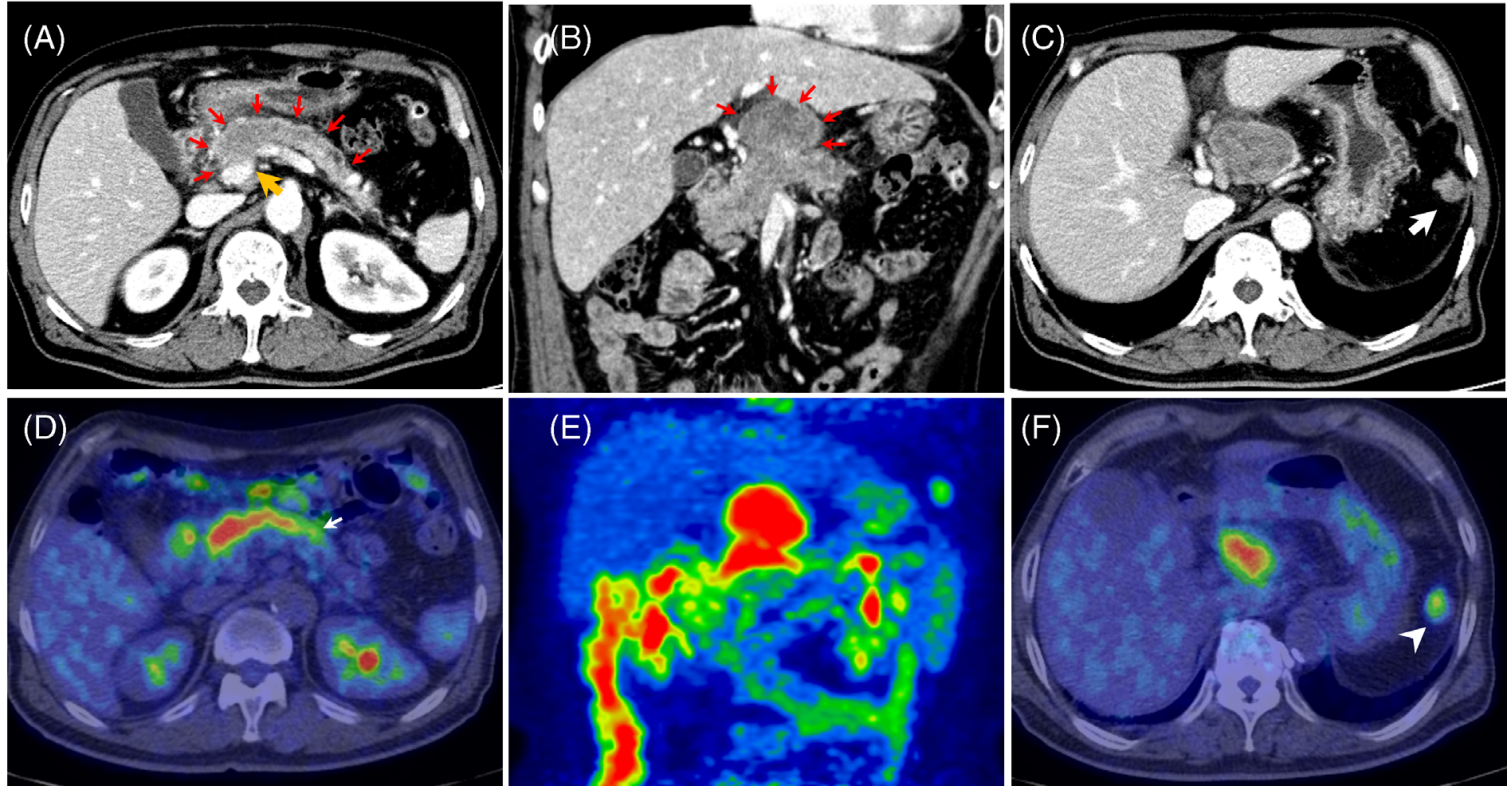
Pre‐treatment images. (A) Abdominal enhanced computed tomography (CT) image showing a pancreatic tumor with growth to the pancreatic body and tail (*red arrows*). The tumor shows slight contact (less than 180° without deformity) with the portal vein (*orange arrow*). (B) Coronal‐view image showing a large tumor with a maximum diameter of 67 mm and remarkable extrapancreatic invasion (*red arrows*). (C) A 23‐mm mass in the left upper abdominal cavity (*arrow*). (D) [^18^F] fluorodeoxyglucose positron emission tomography (FDG‐PET)‐CT showing FDG uptake in the pancreatic tumor (maximum standardized uptake value [SUVmax]: 7.6) with mild FDG uptake in the pancreatic body and tail (*arrow*). (E) Coronal‐view FDG‐PET‐CT image. (F) FDG uptake in the tumor in the abdominal cavity (SUVmax: 3.4; *arrowhead*)
